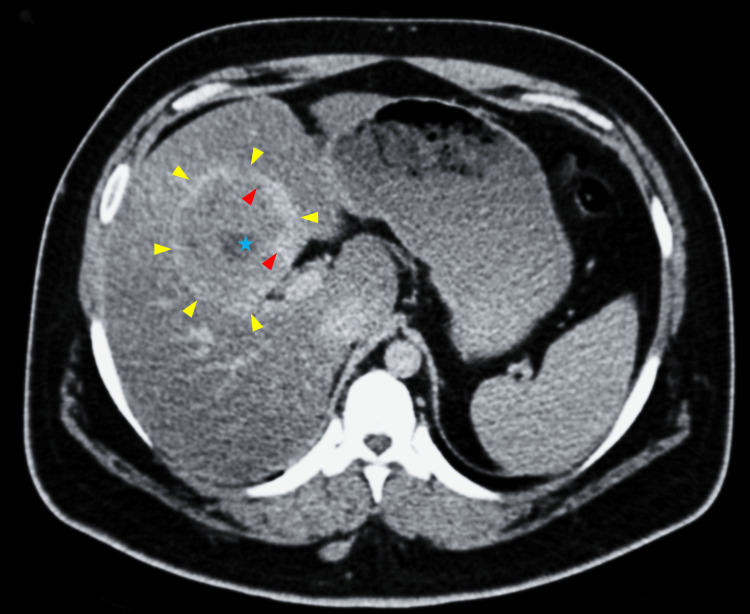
Axial image of intravenous contrast-enhanced CT of the upper abdomen demonstrating a giant cavernous hemangioma (yellow arrowheads) in segment IVb of the liver Interrupted nodular peripheral enhancement (red arrowheads) and a small central scar (blue star) represent atypical imaging features prompting a multidisciplinary review to exclude malignancy.
